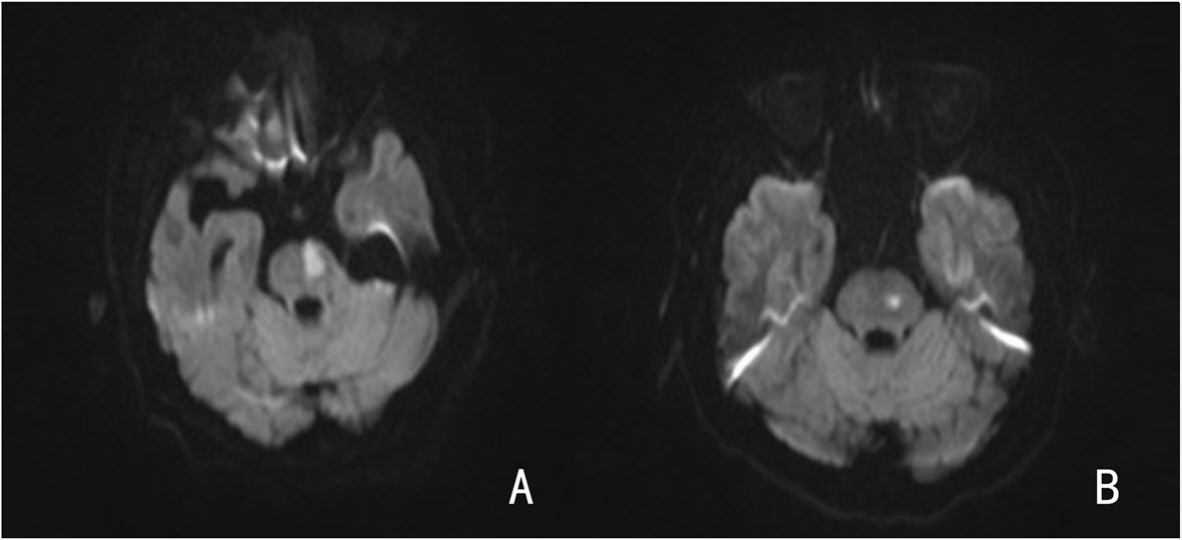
Representative MRI DWI images of patients with BABD (A) and SAD (B)
